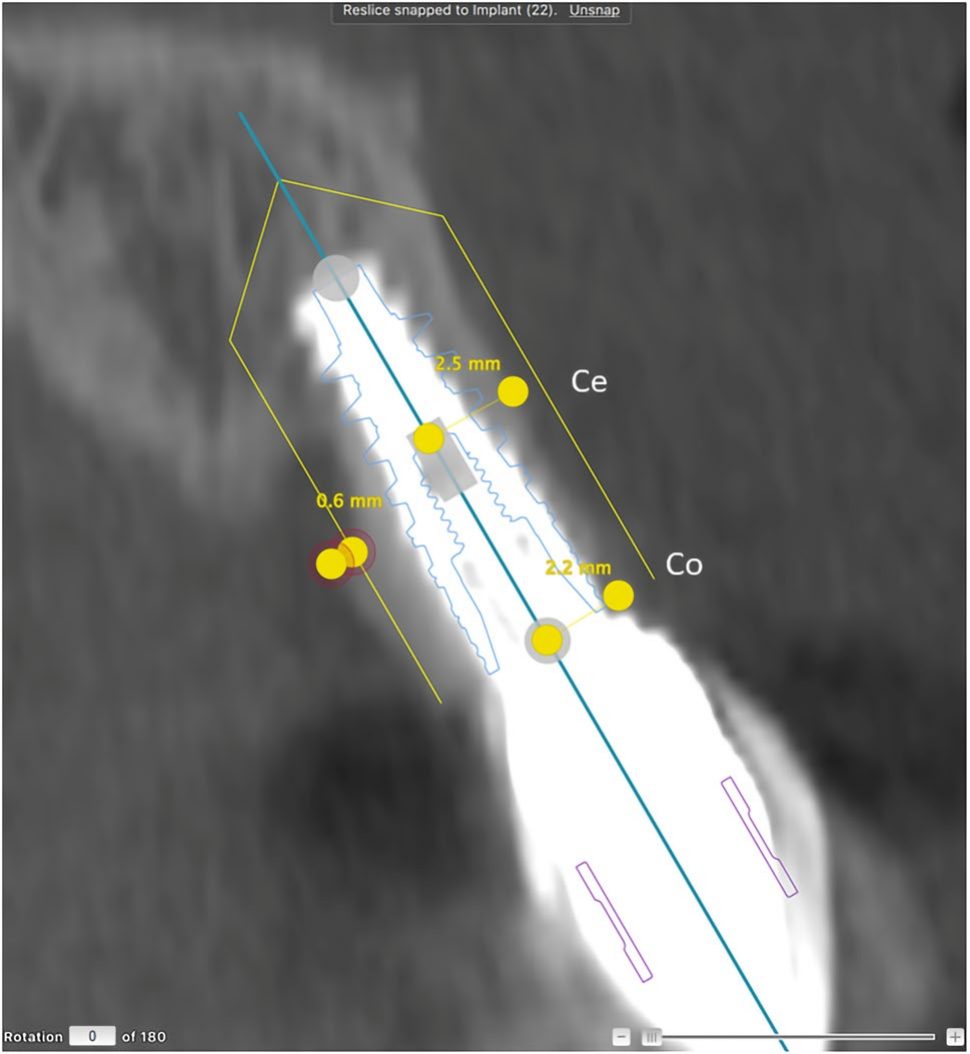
Follow-up CBCT examination in a non-reconstructed patient. The NobelClinician® software planning instrument was superimposed on the actual placement of the implant. Measures of buccal bone were performed at the central (Ce) and coronal (Co) levels of the implant, taking differences between the actual and the planned implant placement into consideration. In this case, there was a sagittal deviation of 0.6 mm between the planned and the actual placement
